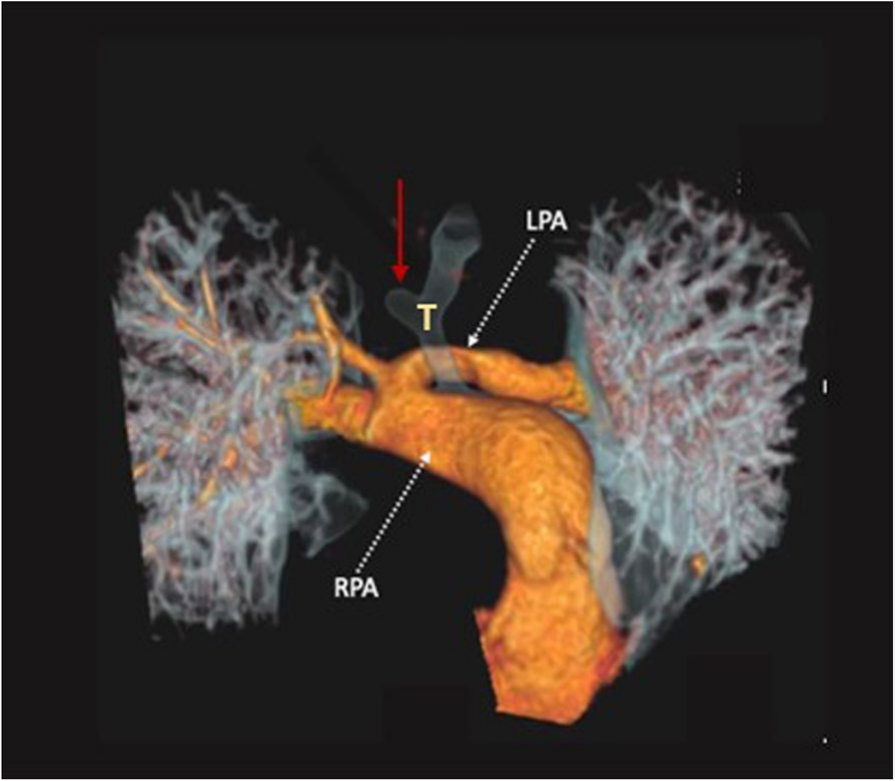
3D volume rendering reconstruction of a cardiac CT in patient with Left Pulmonary arterial sling. The Left Pulmonary Artery (LPA) arises posteriorly and distally from the Right Pulmonary Artery (RPA), coursing posteriorly to the trachea (T). A tracheal bronchus (commonly associated to LPA sling) is also detected (red arrow)
